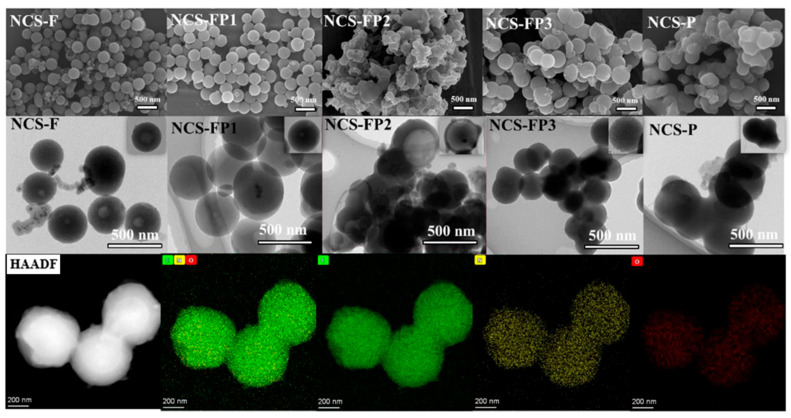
SEM and TEM images of NCS-F, NCS-FP1, NCS-FP2, NCS-FP3 and NCS-P and corresponding NCS-FP3 scanning TEM (C, N, O) energy-dispersive X-ray element mapping.
